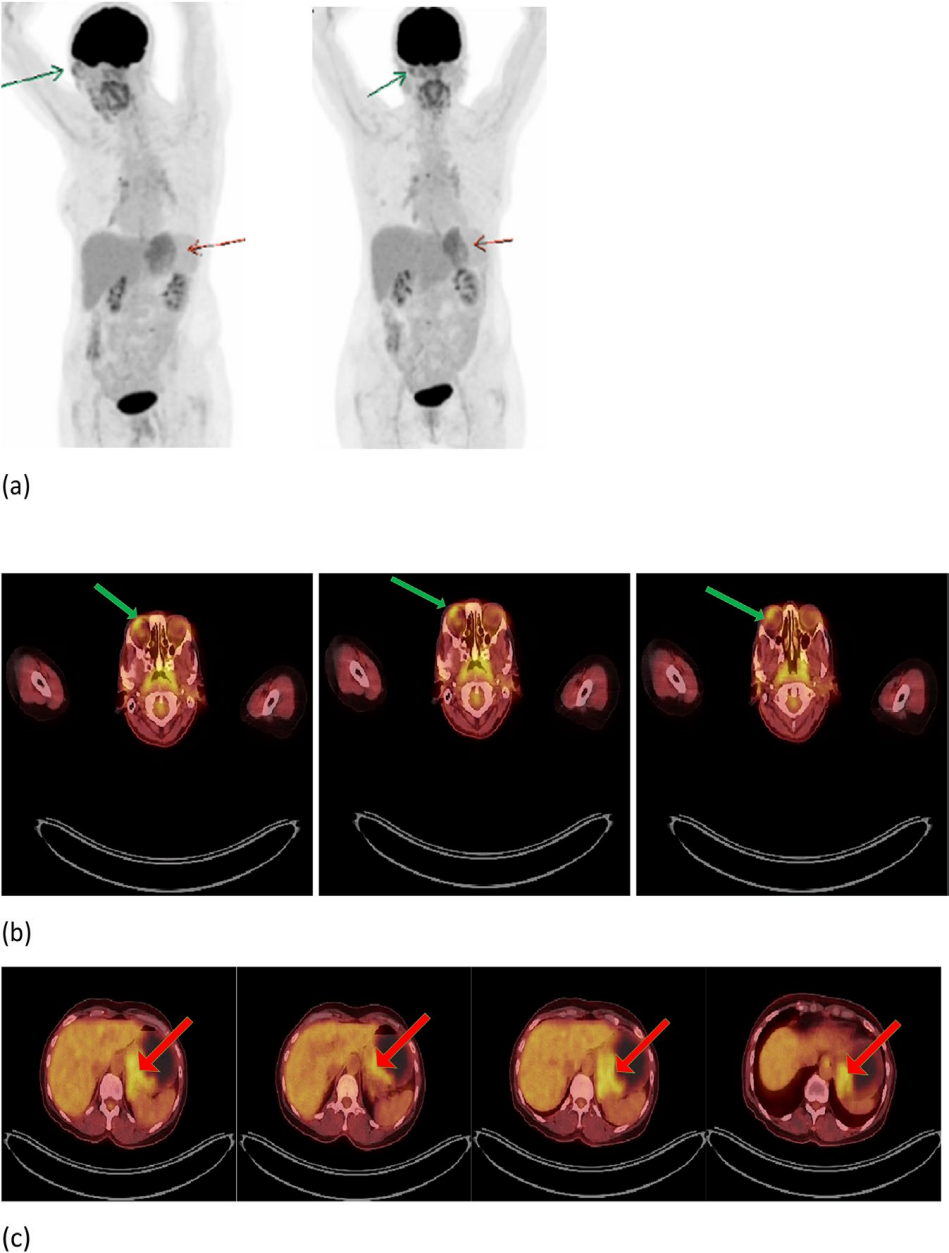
Whole‐body FDG‐PET CT scan after 2 months of chemotherapy. (Panel A): Maximum intensity projection (MIP) PET imaging: The green‐yellow: Orbital lesion. The red‐yellow: The gastric lesion. (Panel B): The green‐yellow show increased metabolic activity in the supralateral aspect of the right orbit. (Panel C): The red‐yellow shows hypermetabolic gastric wall thickening in the fundus and cardia with extension to the lesser curvature, consistent with the residual metabolic activity.
